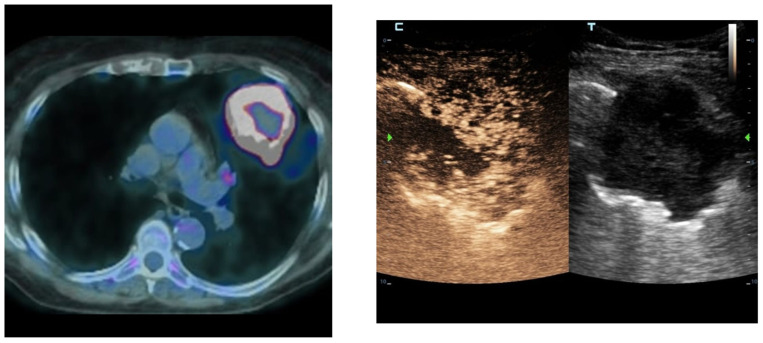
Multimodal imaging of a necrotic lung tumor. Left panel: axial fused positron emission tomography–computed tomography (PET/CT) image showing a hypermetabolic pulmonary mass with a central photopenic area consistent with intratumoral necrosis. Right panel: ultrasound imaging of the same lesion demonstrating a well-defined mass with a hypoechoic central necrotic component, shown on contrast-specific imaging (left) and corresponding B-mode ultrasound (right).
